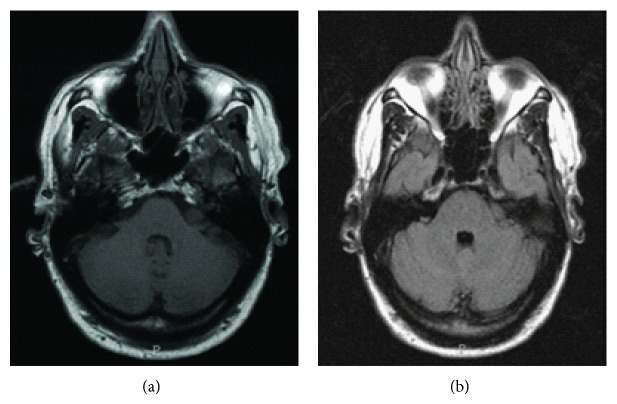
(a) T1-axial MRI with contrast and (b) T2-axial Flair MRI performed at initial encounter demonstrate a minimally enhancing mass in the left cerebellopontine angle.
